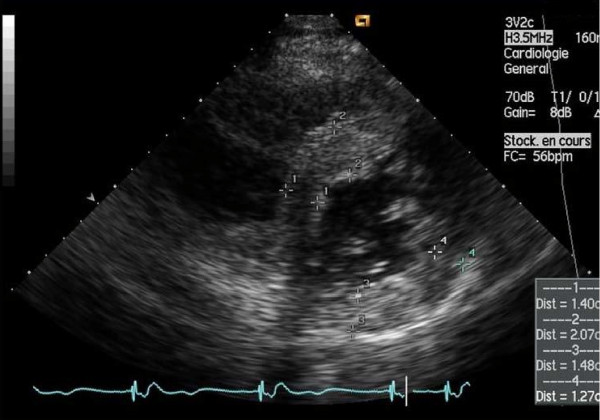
Echocardiography: parasternal short axis showing left ventricular hypertrophy. Courtesy: Pr Albert A. HAGEGE, Université René Descartes, Paris, France.
